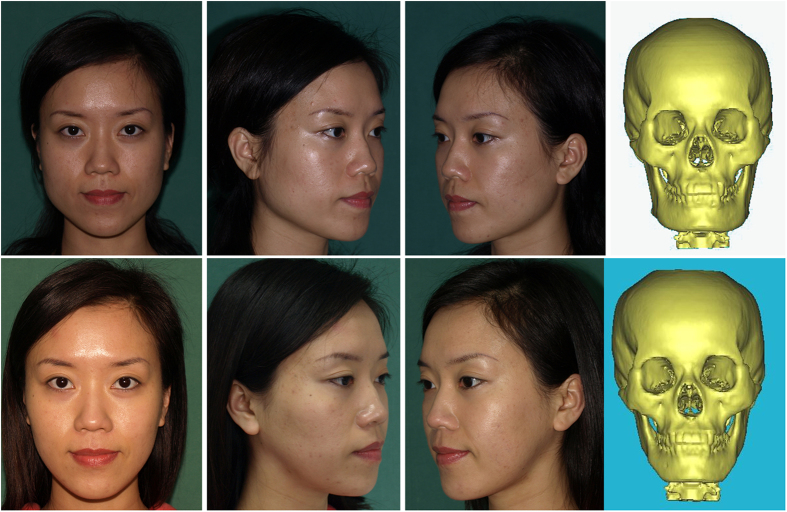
Photographs and computed tomography (CT) scans before surgery (above) and four months after surgery (below) revealed a satisfactory outcome of the treatment for mandibular angle hypertrophy.
